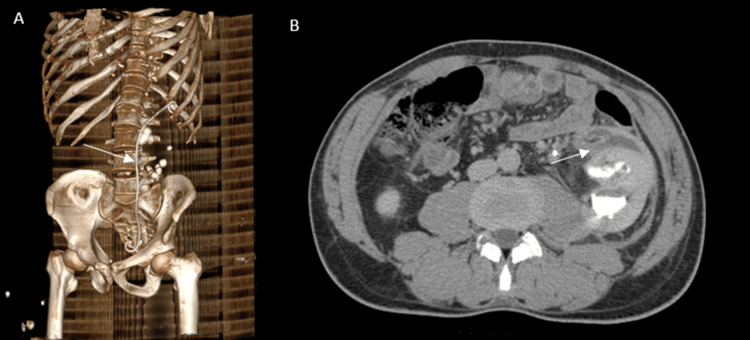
CT images after placement of the double-J stent (A) Three-dimensional volume-rendered reconstruction from the follow-up CT showing a double-J ureteral stent in situ; (B) Axial CT image in the venous phase demonstrating perirenal fluid collections with peripheral enhancement.
